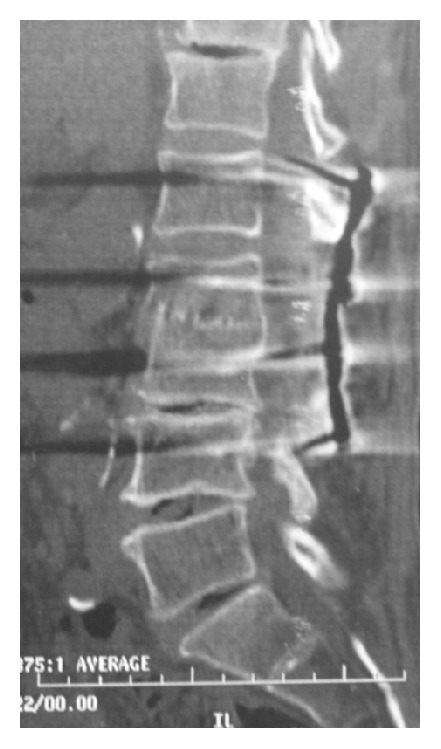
CT scan at the 5-year followup confirming bony union achievement and a solid and stable fusion of the spine (Case 1).
